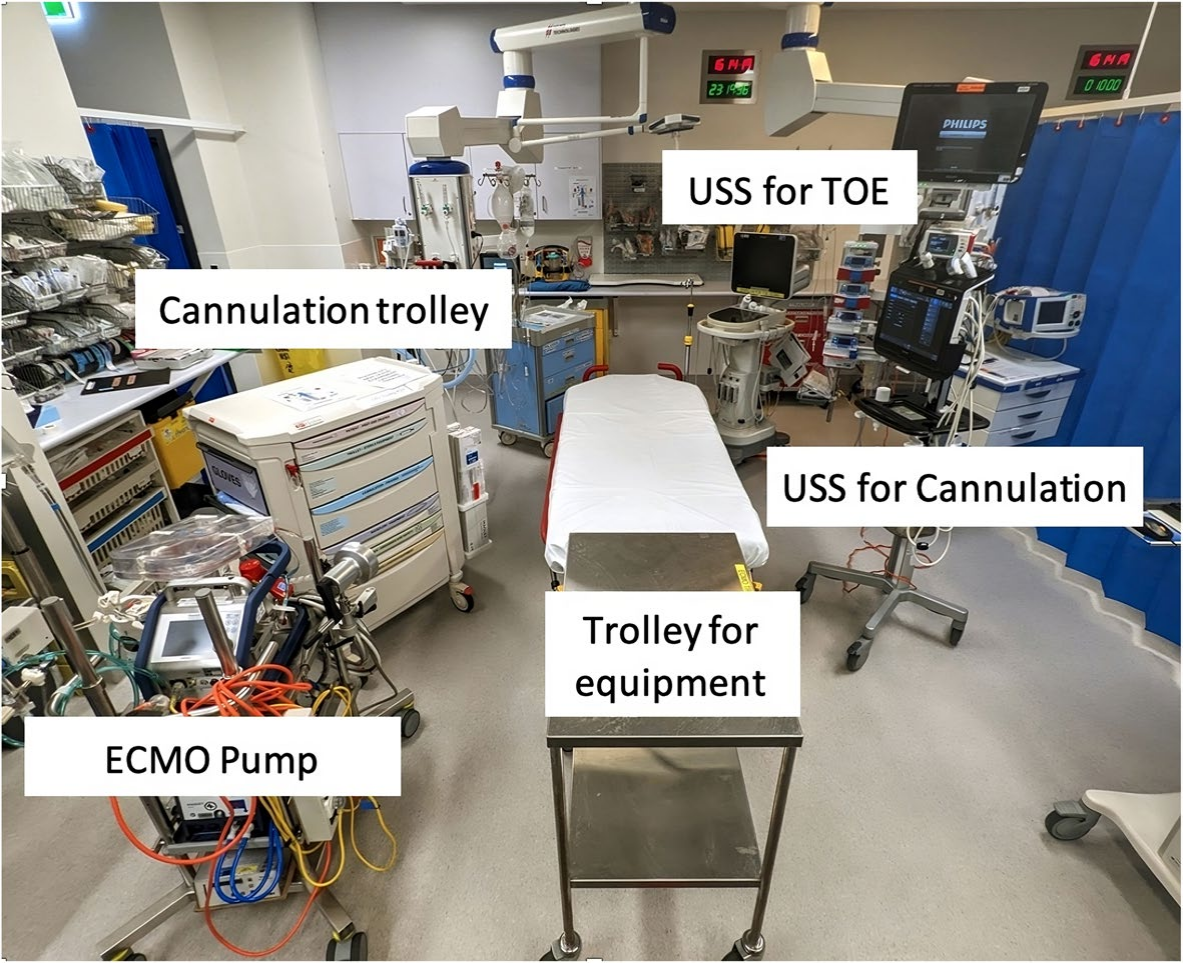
Layout of the emergency department resuscitation bay for ECPR. US = Ultrasound, TOE = Transoesophageal Echocardiography
